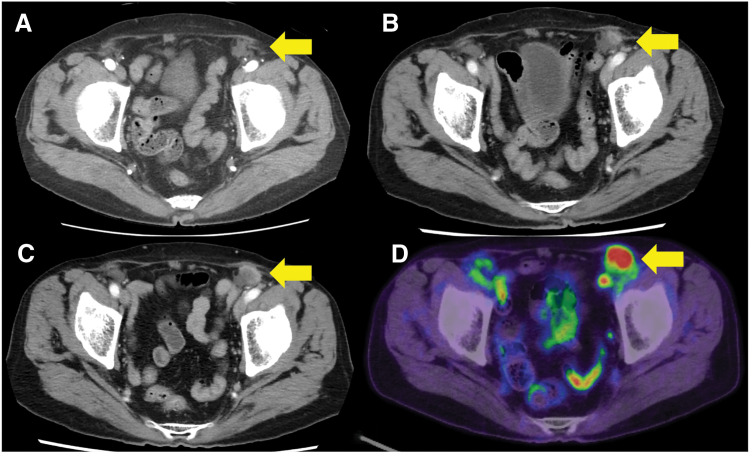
CT and PET–CT findings. (A) Preoperative contrast-enhanced CT for transverse colon cancer revealed only postoperative changes from prior inguinal hernia repair. The arrow highlights the absence of apparent metastatic nodules. (B) Contrast-enhanced CT at 6 months postoperatively demonstrated the appearance of an enhancing nodule in the left inguinal region. The arrow highlights the inguinal mass. (C) Contrast-enhanced CT at 1 year postoperatively showing interval enlargement of the left inguinal lymph node. The arrow highlights the inguinal mass. (D) PET-CT revealing fluorodeoxyglucose uptake in the left inguinal lymph node. The arrow highlights the inguinal mass.
